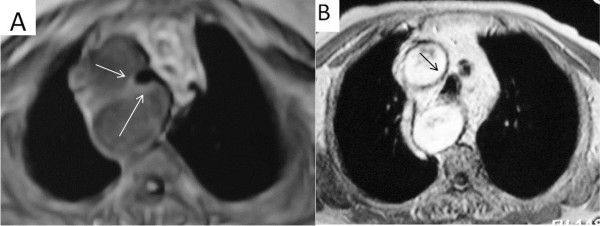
Thoracic MRI and CT images; Thoracic CT and MRI images showing marked compression of the trachea in patients with hypertension and RSAA (arrows).
